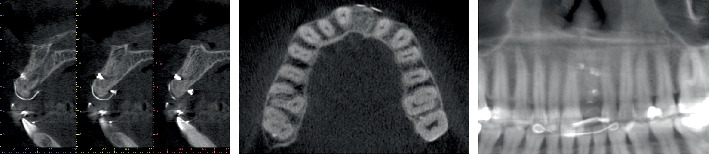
CBCT control at 9 months after bone augmentation. Tangential, axial, and panoramic CBCT images of the site.
